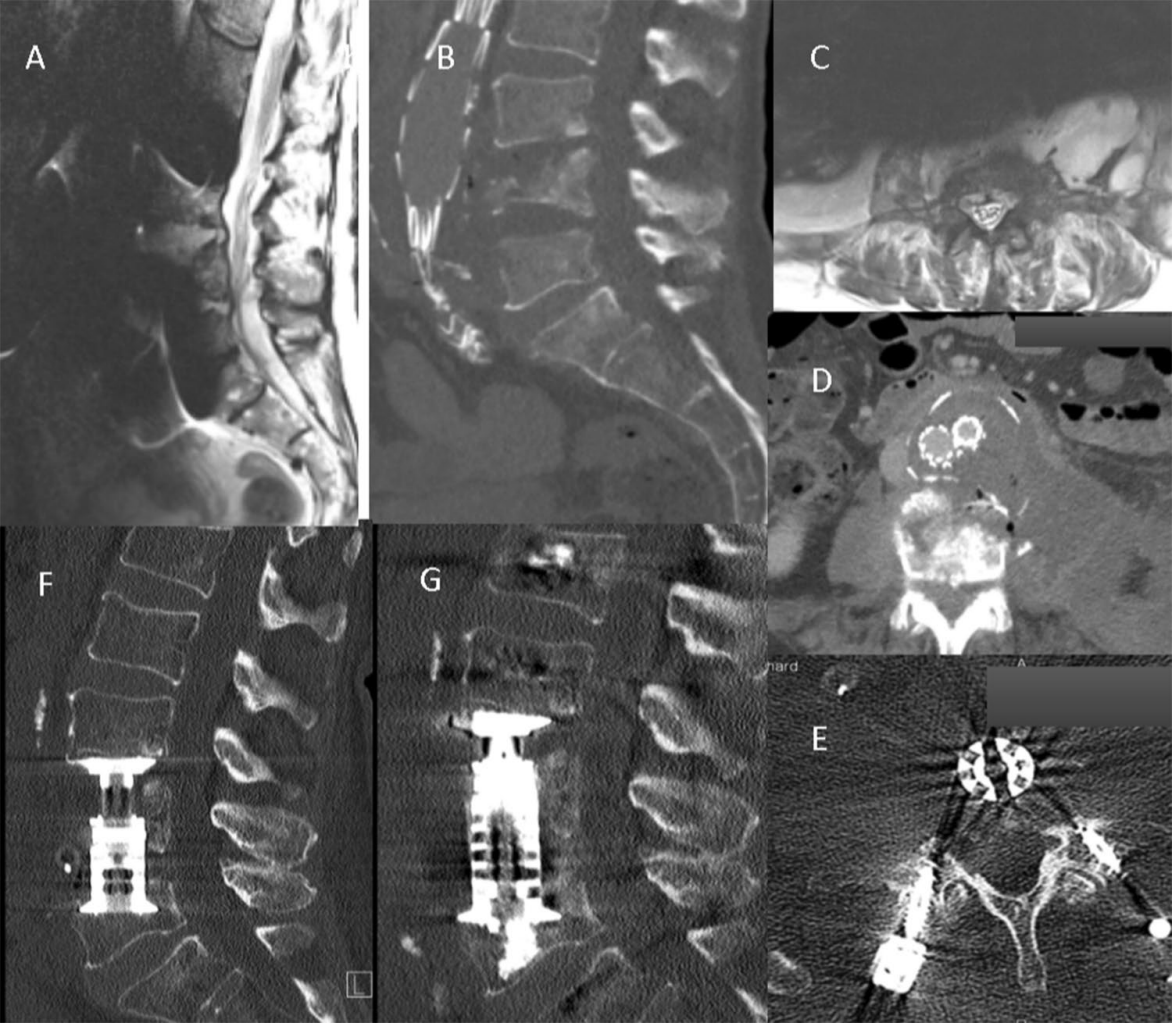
a, c Magnetic resonance imaging (MRI) with endovascular aortic repair (EVAR) in place and large abscesses. b, d Vertebral body destruction with *per continuitatem* infection to the vessel prothesis. f Computed tomography (CT) scan showing the loss of height of L5 with revision surgery from posterior in (e, g)
